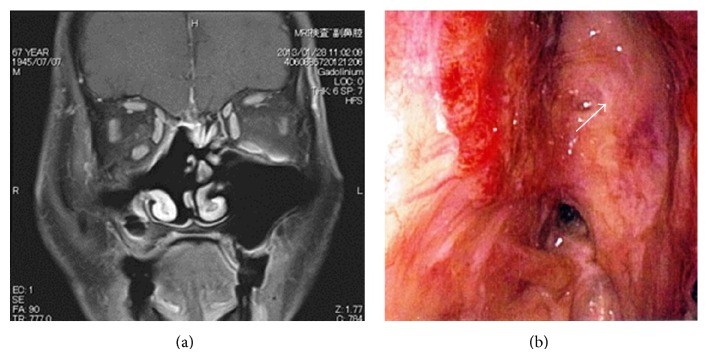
Postoperative MRI and local finding in case 2. (a) T1-weighted coronal image (gadolinium+) showing no recurring mass. (b) The white arrow shows the pedicled nasal septal flap in the right nasal cavity.
